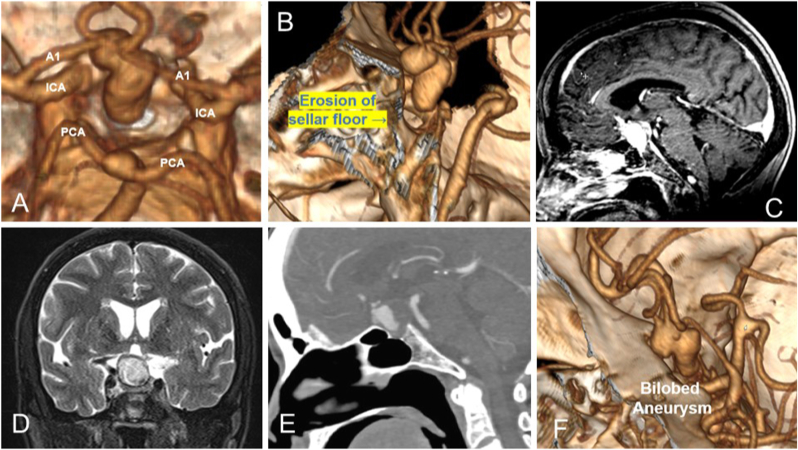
A- 3D reconstruction of aneurysm extending into the sella with vessels of circle of willis. B- 3D reconstruction of angiogram at the midsagittal level with aneurysm extending into the sella. C- T1 post contrast MRI showing enhancing mass in the sella. D- T2 hyperintense mass in the sella. E− CT angiogram showing sellar floor defect. F- bilobed morphology of the aneurysm extending into the sella.
